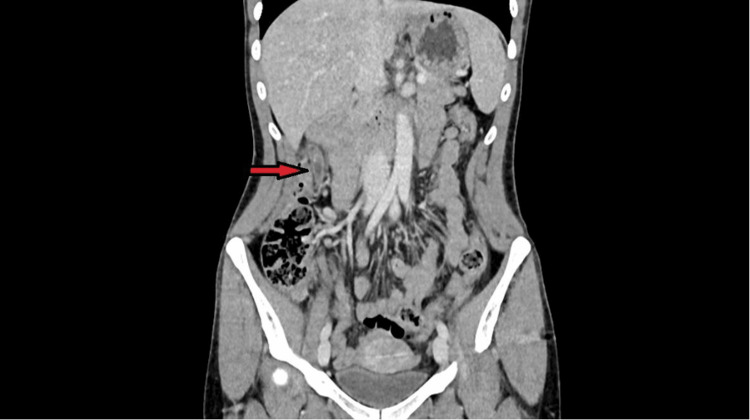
Coronal CT image showing the tip of the appendix located in the subhepatic region.
